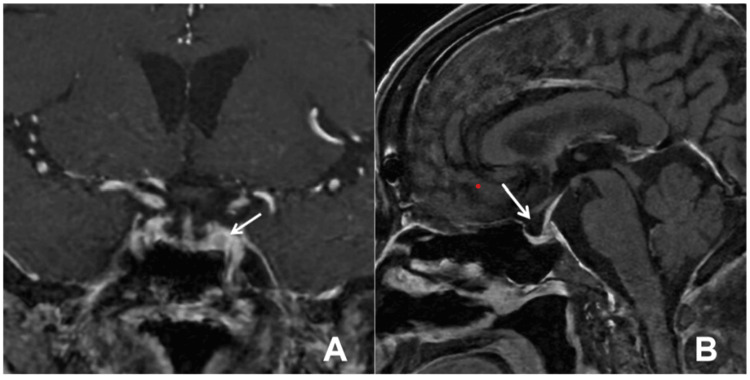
High-resolution MRI images of the patient's pituitary gland. 4A depicts a round-like T1-phase low-signal adenoma located on the left side of the pituitary gland, while 4B shows a sagittal view of the vacuolated sella turcica.
